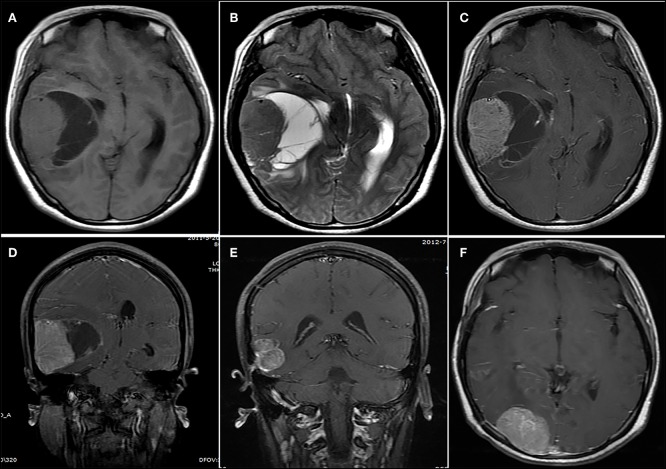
A lesion showing a concomitant cystic and solid appearance. (A,B) The lesion showed an isointense signal on (A) the T1-weighted image and (B) the T2-weighted image. (C) Contrast-enhanced axial and (D) coronary images show significant heterogeneous enhancement. (E,F) Fourteen months after initial surgery, (E) tumor recurrence and (F) metastasis were observed.
